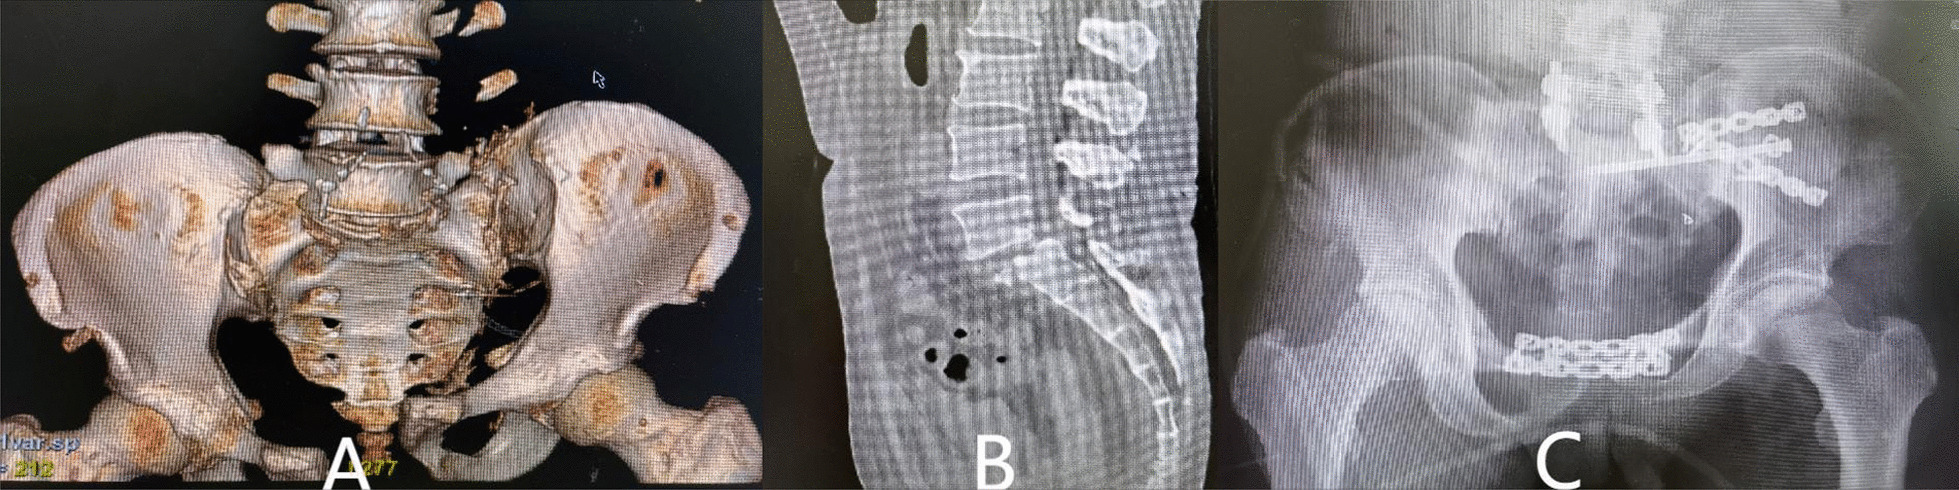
Case 15. A 52-year-old man who was suffered from compression injury underwent lumbosacral fixation and S1 sacroiliac screw fixation for a 301SPD type IIIa fracture. A Preoperative X-ray shows bilateral sacroiliac joint injury. B Preoperative CT scan cross-sectional image shows displacement of fracture. C The X-ray image shows that the fracture was fixed satisfactorily
